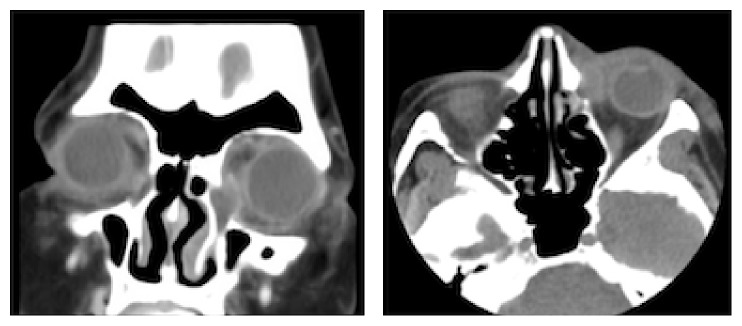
Coronal and axial CT of the orbits with contrast showing an enlarged left lacrimal sac and preseptal inflammatory changes consistent with acute dacryocystitis with preseptal cellulitis.
